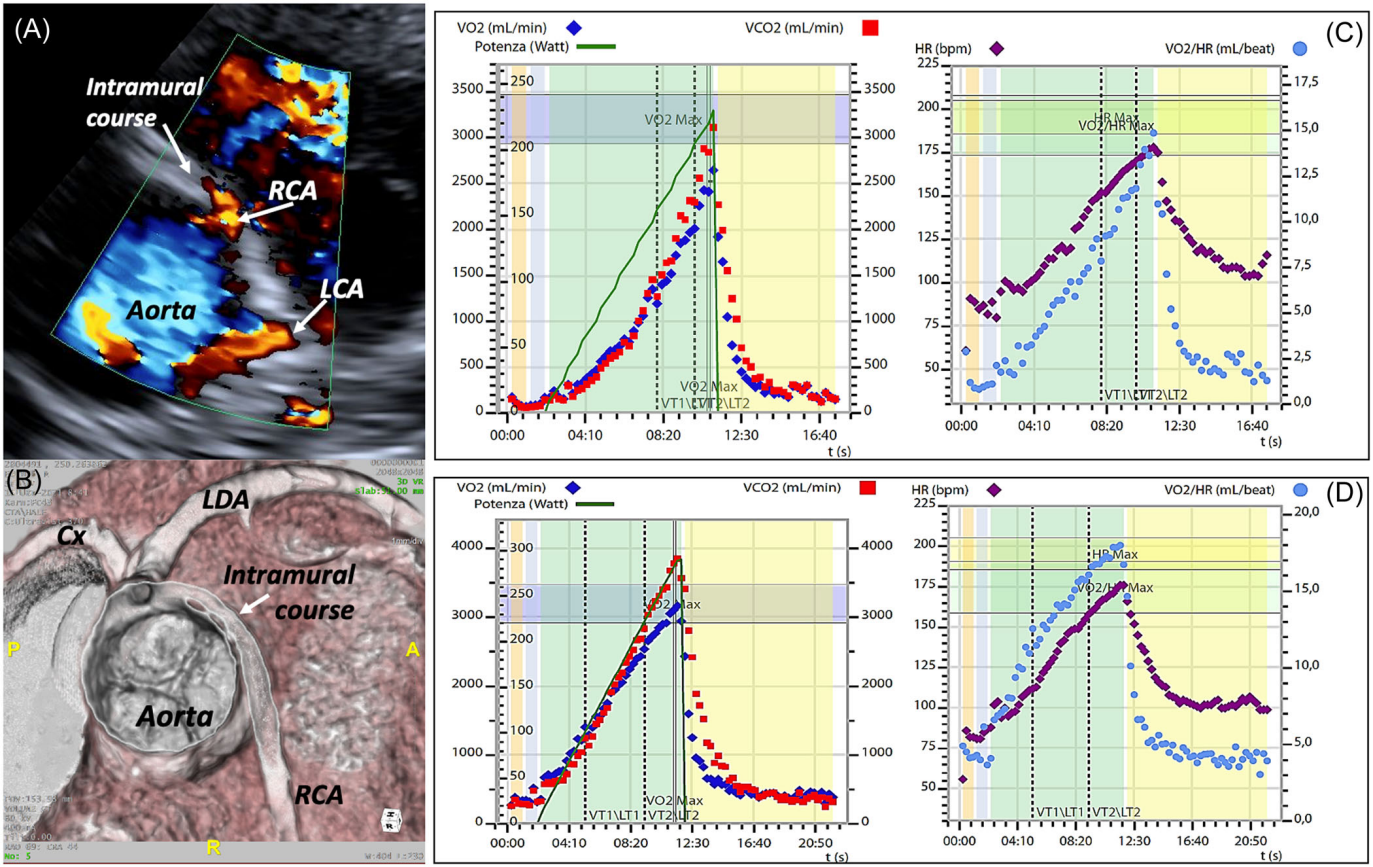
Fourteen years‐old competitive soccer player with presyncope and cold sweating during an official competition. At the bloodwork in the hospital, increased levels of high‐sensitive Troponin were documented. The transthoracic echocardiogram (A) suspected an anomalous origin of the right coronary artery coursing between the aorta and pulmonary artery. The computed tomography angiography (B) confirmed the suspicion, showing an intramural course of the anomalous vessel. Due to the symptoms and imaging risk features, surgery was offered, and the young patient underwent unroofing procedure. Panel (C) shows the cardiopulmonary exercise test (CPET) results before surgery. The oxygen uptake (VO_2_) at peak of the exercise was 37.2 mL/kg/min (73% of the expected) and the oxygen pulse (heart rate/VO_2_) was 14.2 mL/bpm (84% of the expected). Three months after unroofing procedure, following a light aerobic rehabilitation protocol, the CPET results (D) showed an increase of both VO_2_ peak (46.1 mL/kg/min, 91% of the expected) and oxygen pulse (16.9 mL/bpm, 105% of the expected), falling in the normal range for this boy. Cx, circumflex coronary artery; LAD, left anterior descending artery; LCA, left coronary artery; RCA, right coronary artery.
